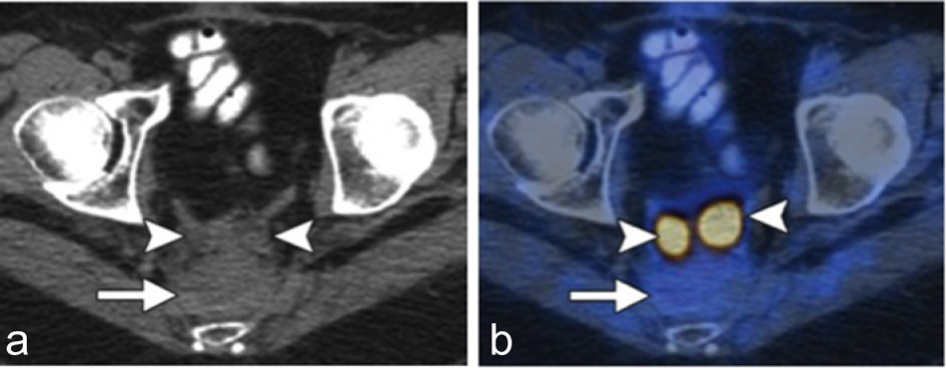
26-year-old female with Grade 2 SCC of the cervix, following radical TAH BSO and EBRT. Surveillance CT (a) demonstrates that non-specific changes in the pre-sacral space (arrow) and hysterectomy bed (arrow heads) and recurrence would be indistinguishable from radiotherapy changes. (b) Demonstration of FDG avid biopsy confirmed recurrent disease in the hysterectomy bed (arrow) and no recurrence in the pre-sacral space (arrow heads). EBRT, external beam radiotherapy; FDG, fluoro-2-deoxy-D-glucose; SCC, squamous cell carcinoma.
